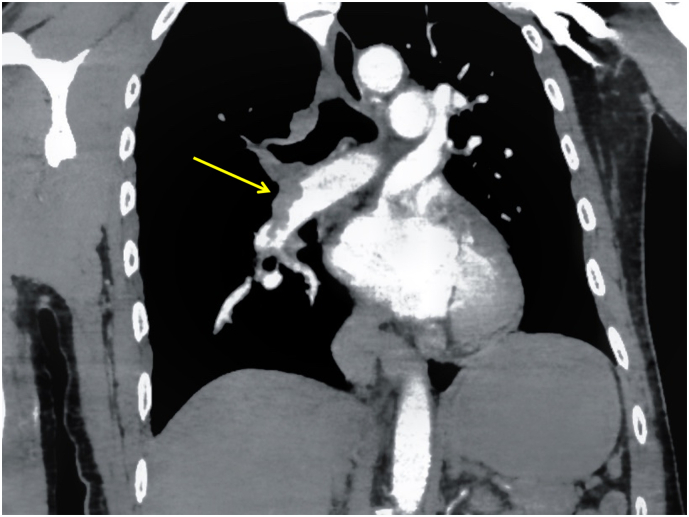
After thrombolytic therapy, CTA finding (T+ 1:36) there is still filling defects clots in the right upper lobe artery and multiple branches of the pulmonary artery.
